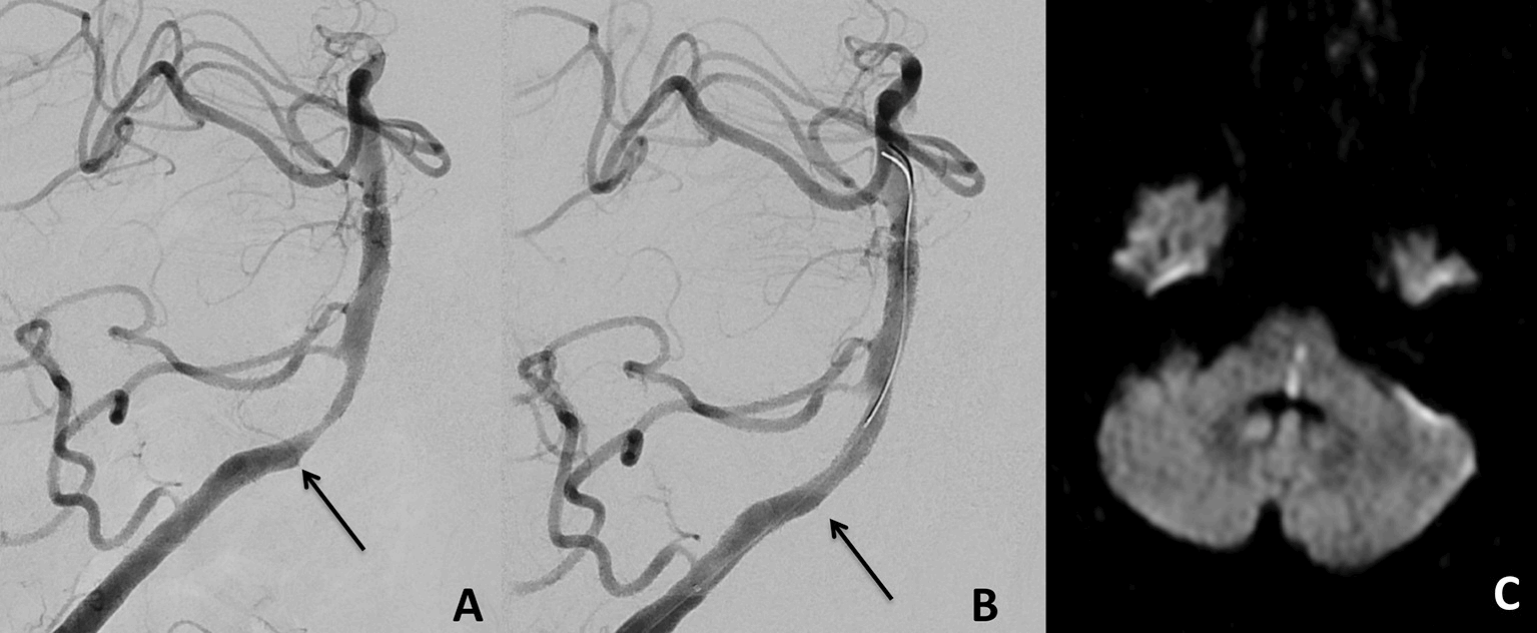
Proximal basilar artery stenosis before treatment (A) with the arrow indicating a perforating artery arising at the proximal part of the stenosis. Stent-PTA led to an occlusion of this perforating artery (B). MRI at day 1 after the procedure showed a left paramedian pontomedullary DWI lesion leading to a mRS score of 4 on follow-up examination after 6 months (C).
